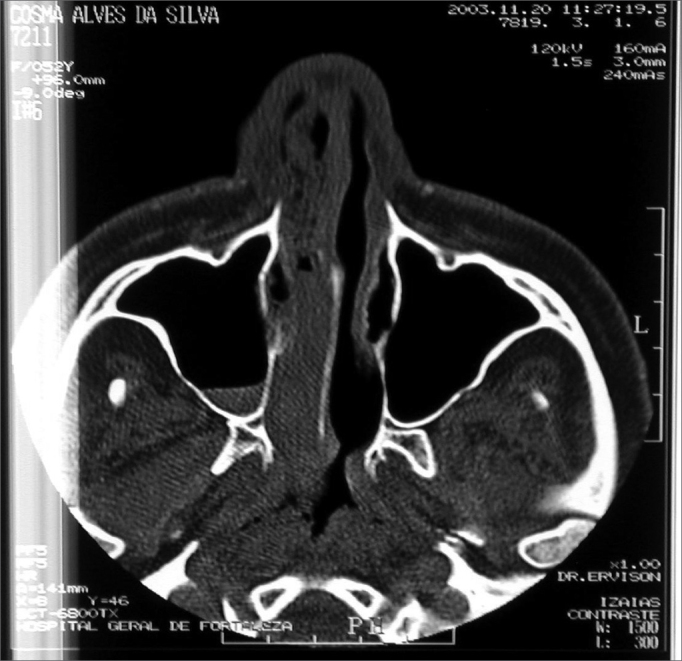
CT scan - axial view of Extramedullary Plasmocytoma (case 3).
